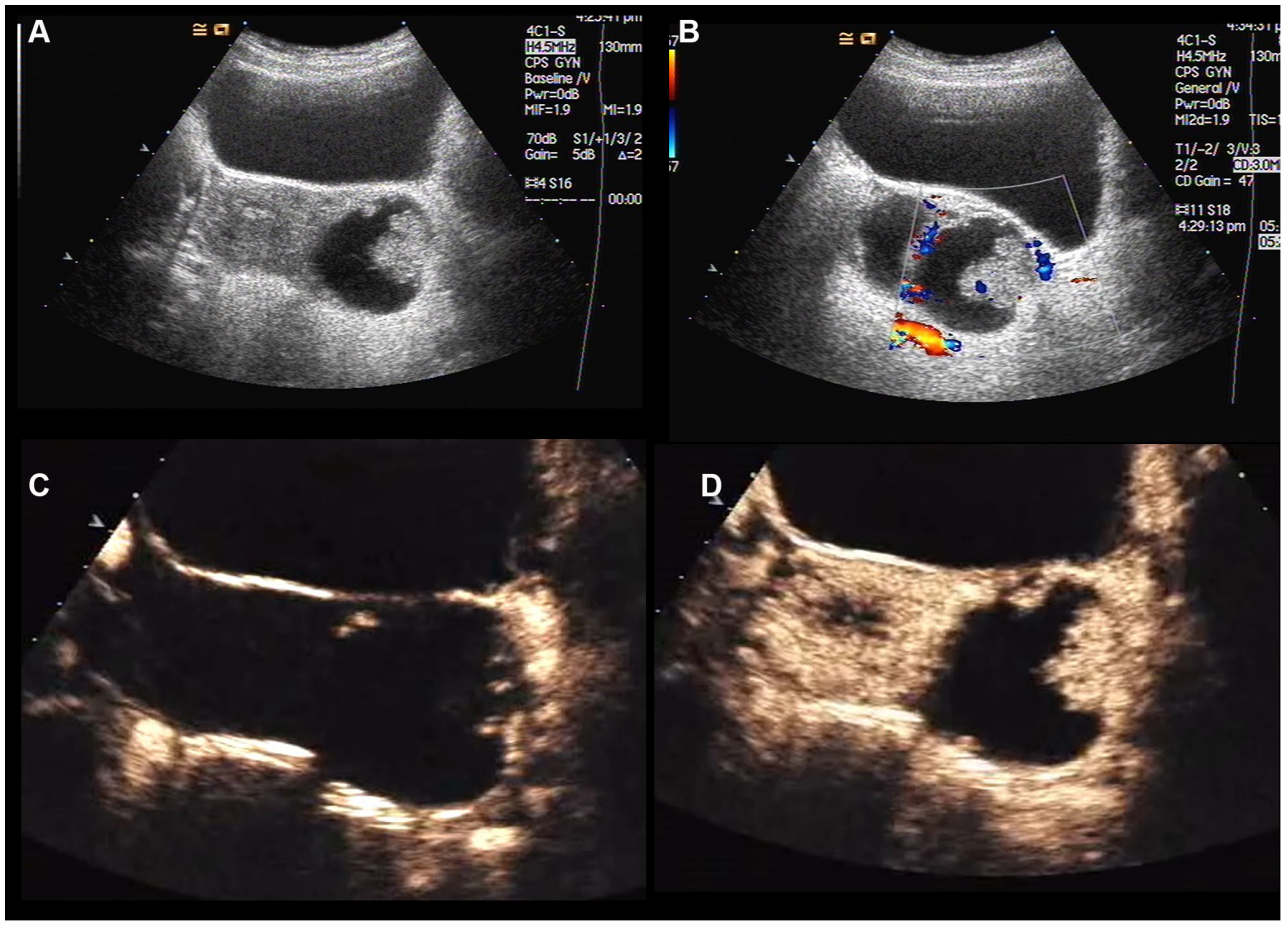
A 22-year-old woman with serous cystadenocarcinoma. A: Gray-scale sonogram shows an 5.2-cm echogenic mass (arrow) with solid projection at the left of uterus. B: Color Doppler shows minimal-moderate vascularization. C: CEUS scan at 12 s shows earlier enhancement (arrow) in comparison with myometrium. D: CEUS scan at 15 s shows inhomogeneous hyper-enhancement with agent perfusion inside (arrow).
